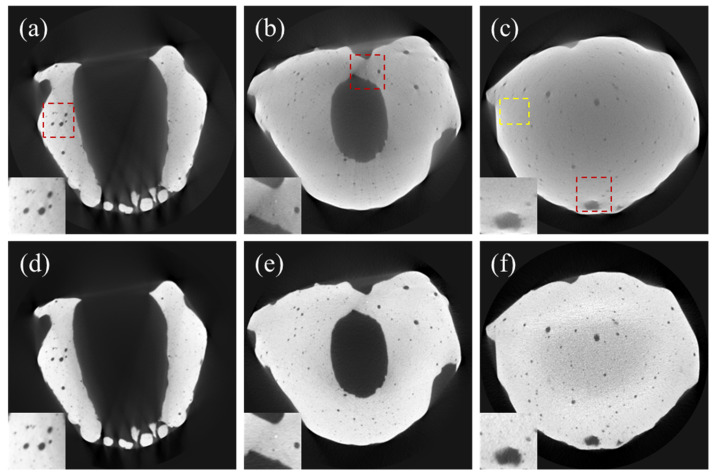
CT images of Model 2. (a–c) Uncorrected CT images. (d–f) DSC-SAC-corrected CT images. The zoomed images in the bottom left corner are related to the red ROIs while the yellow Roi is used to evaluate the signal to noise ratio (SNR).
